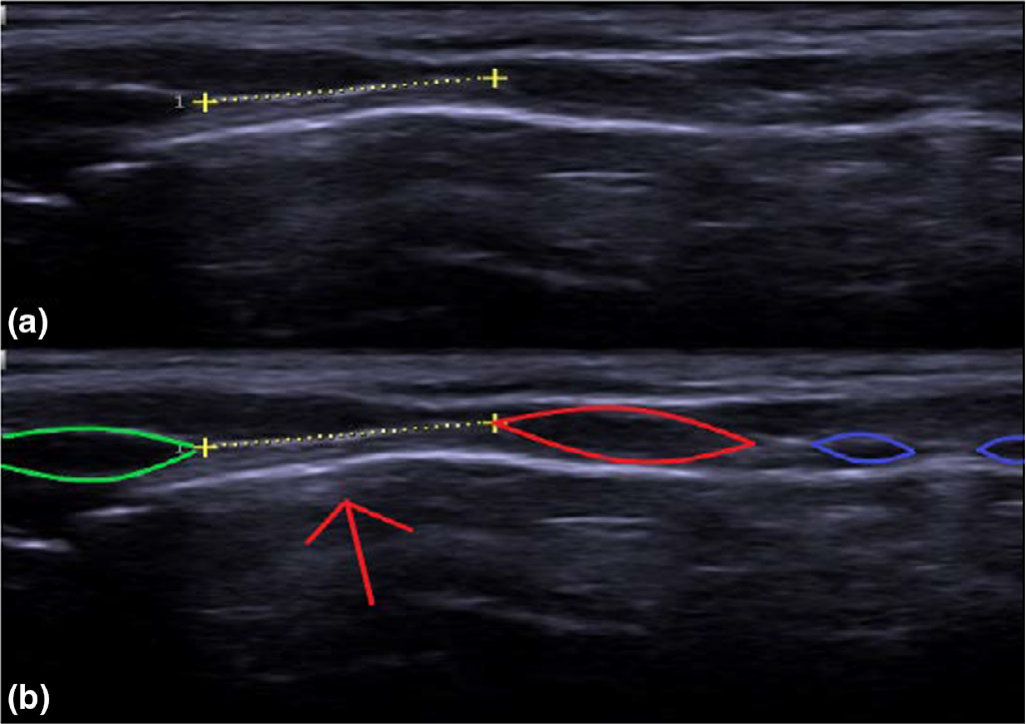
(a) Ultrasound image showing the longitudinal view of the CTM. (b) The CTM is seen as a white horizontal line formed at the mucosa–air interface (red arrow). Tracheal rings are shown in blue ovals. Cephalically, the CTM is bound by the inferior edge of the thyroid cartilage (green oval), while caudally, the CTM is bound by the superior border of the cricoid cartilage (red oval). The length of the CTM is measured between these borders.
